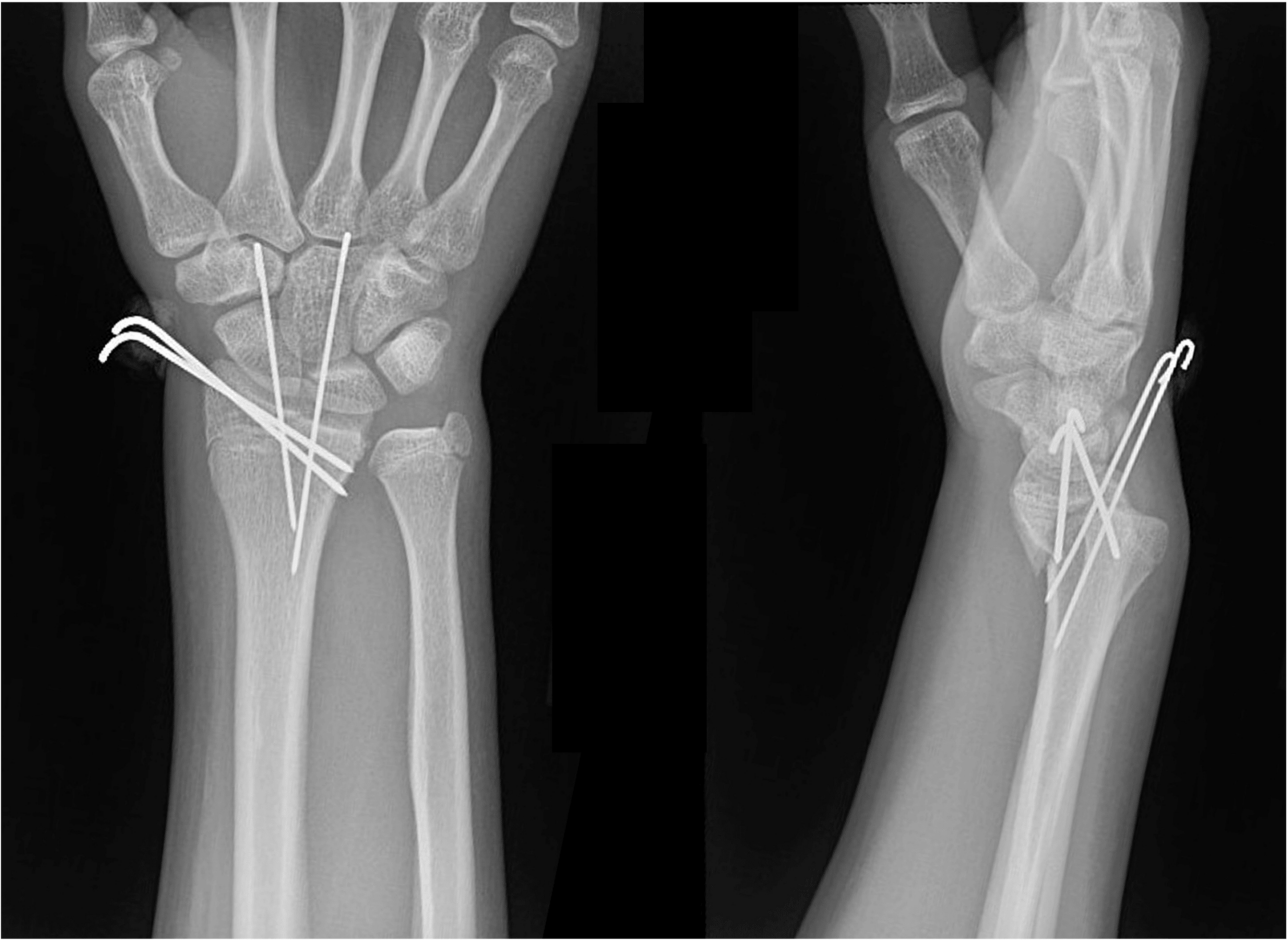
Follow-up radiographs Postoperative radiographs demonstrating loss of fracture reduction at first postoperative follow-up appointment.
